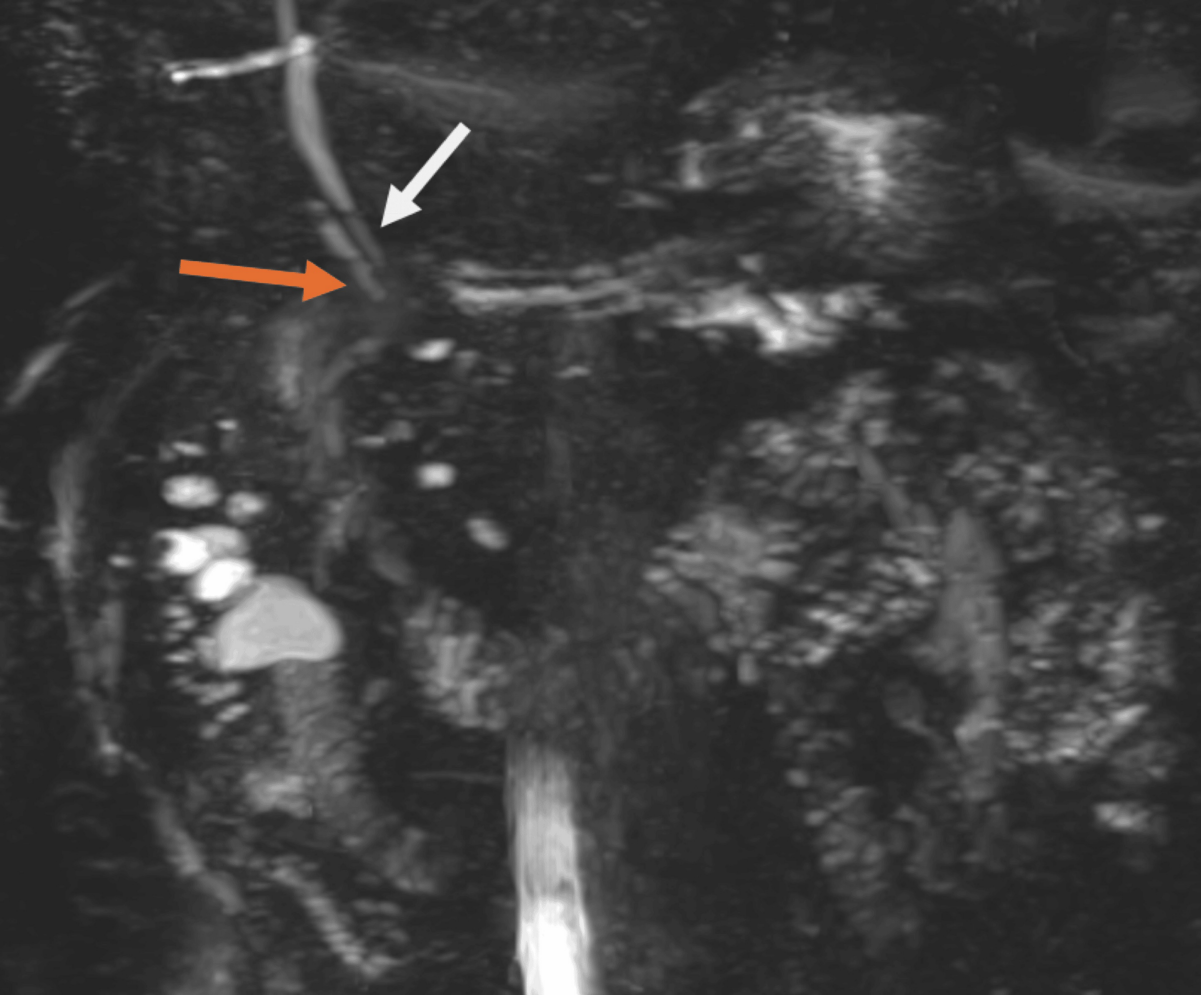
An T2-weighted MRCP SPACE sequence was used to visualize biliary tree. The white arrow indicates the CBD, and the orange arrow demonstrates the cystic duct coursing parallel to the CBD and inserting near the ampulla of Vater. MRCP: magnetic resonance cholangiopancreatography; CBD: common bile duct
